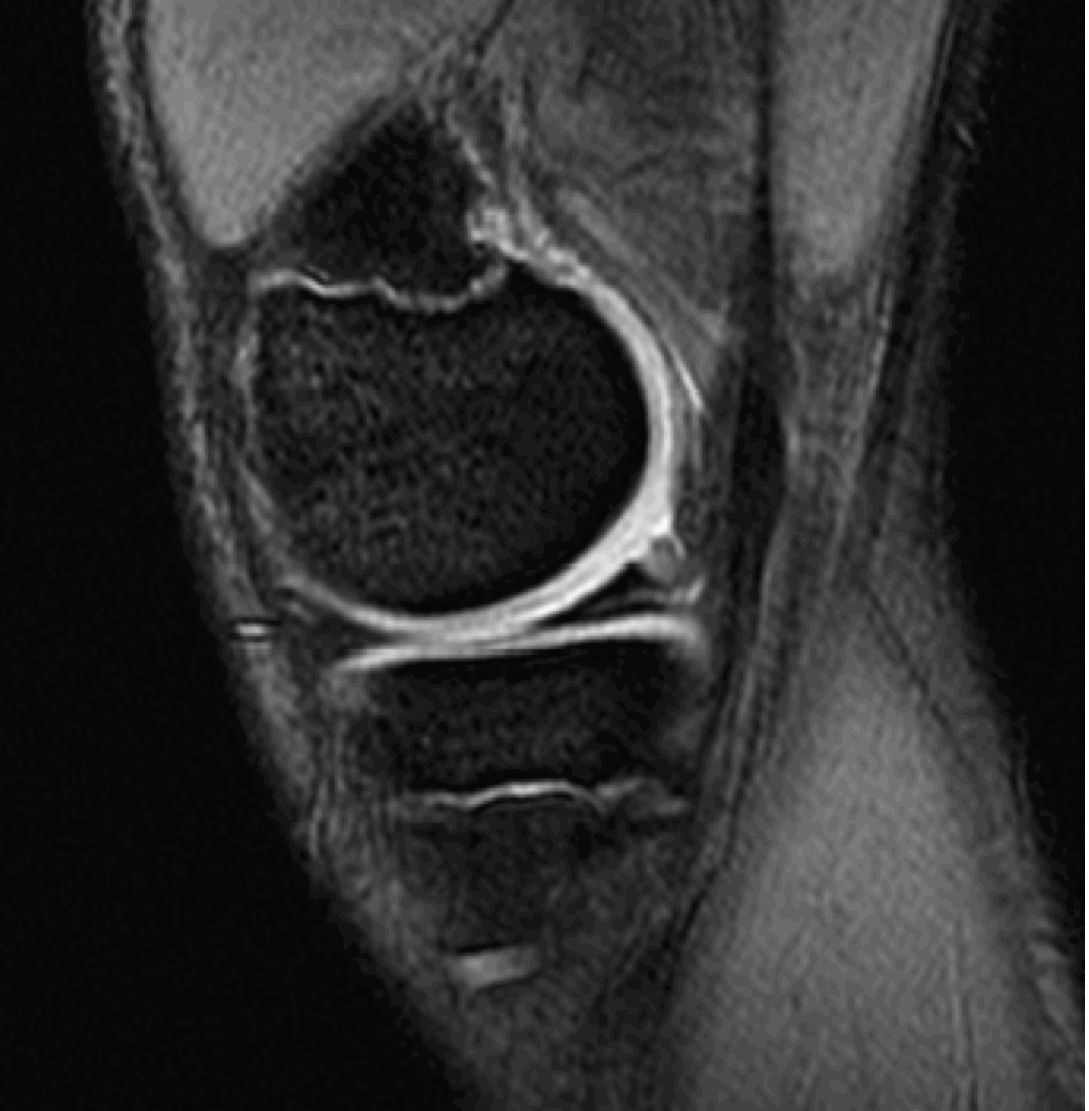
T2 weighted Saggital MRI showing the medial meniscus.
